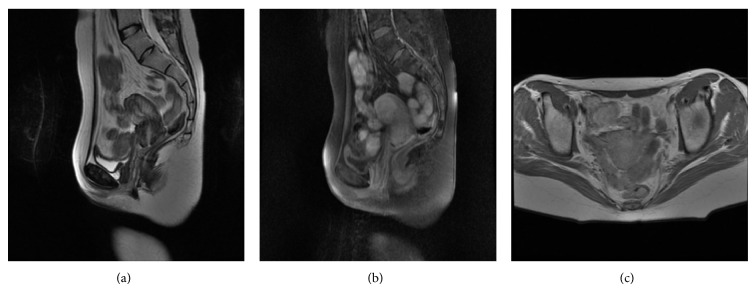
(a) Sagittal T2-weighted, (b) sagittal postcontrast T1-weighted fat suppressed, and (c) axial postcontrast T1-weighted images demonstrate complete resolution of the mass.
